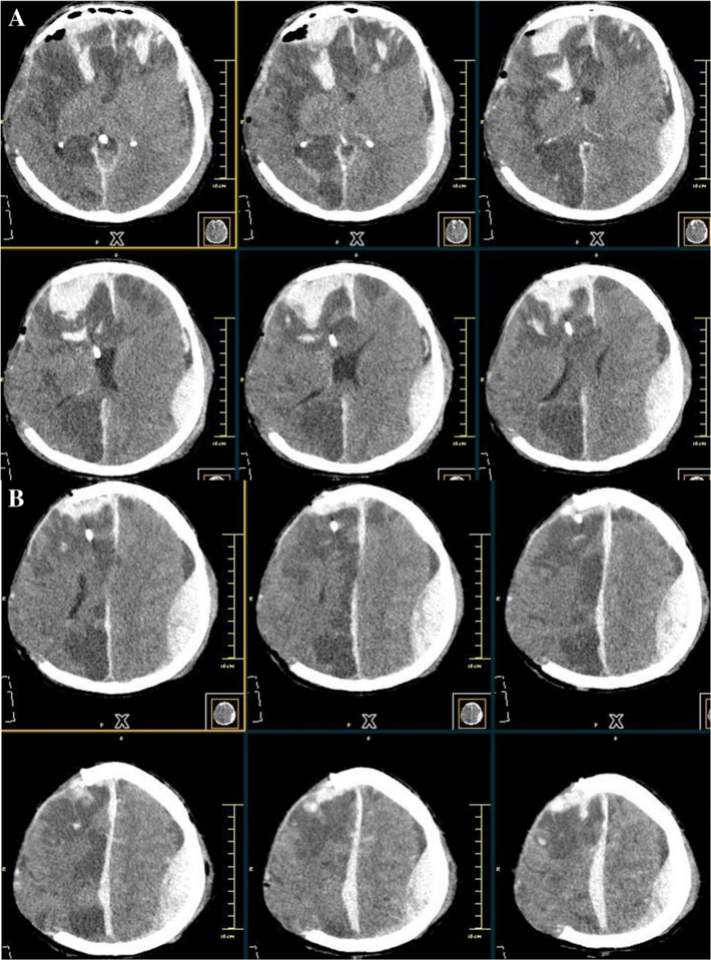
(A/B): Computed tomography following decompressive surgery showing contralateral extradural hematoma.
